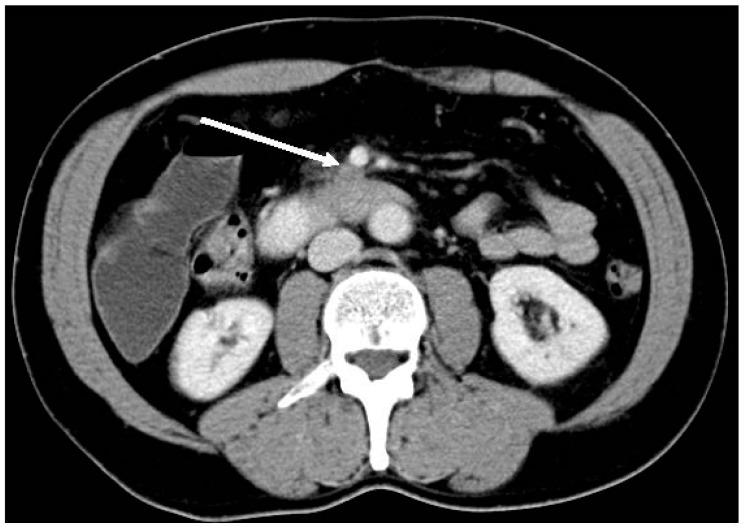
Computed tomography image of a tumor in the uncinate process with tumor contact to the superior mesenteric vein (smv) over a distance of about 2 cm (arrow: “borderline resectable”).
